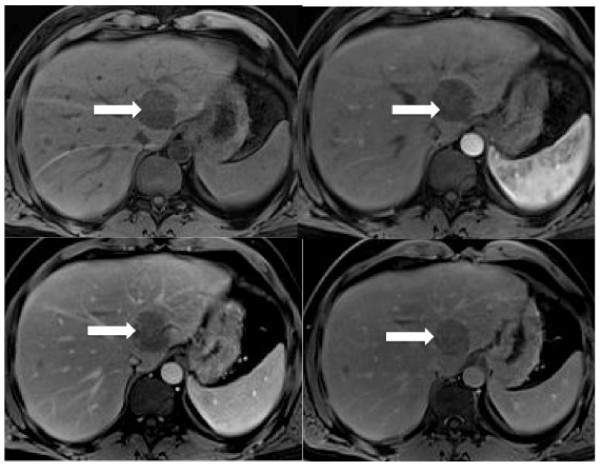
T1-weighted VIBE sequences obtained before and after contrast median injection showed a poor enhancement within the solid lesions (white arrows).
